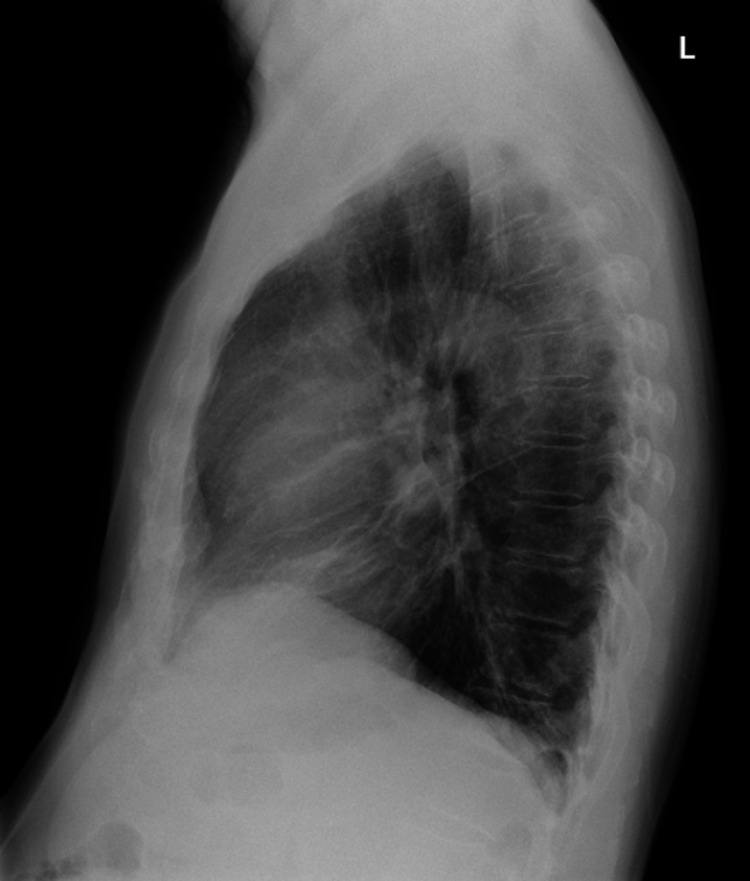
Lateral chest radiograph of anterior mediastinal mass
